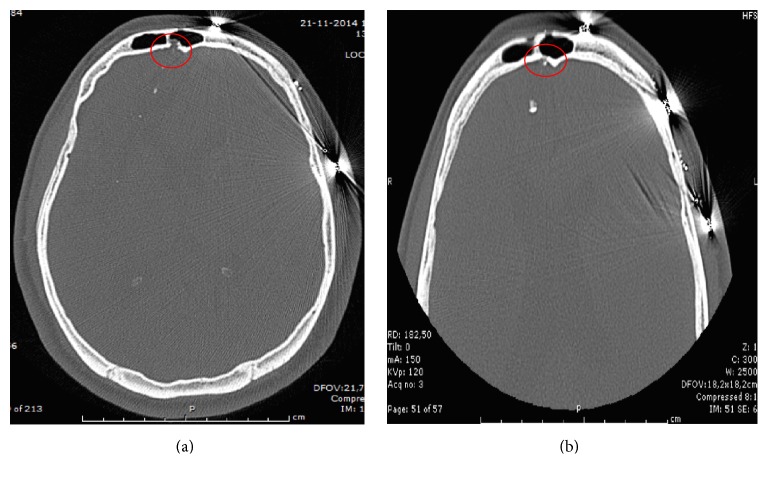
Case 3. (a) Preoperative CT scan showing a CSF leak in the posterior wall of the frontal sinus. (b) Seven-month postoperative CT showing a less dense bony closure of the surgical defect.
